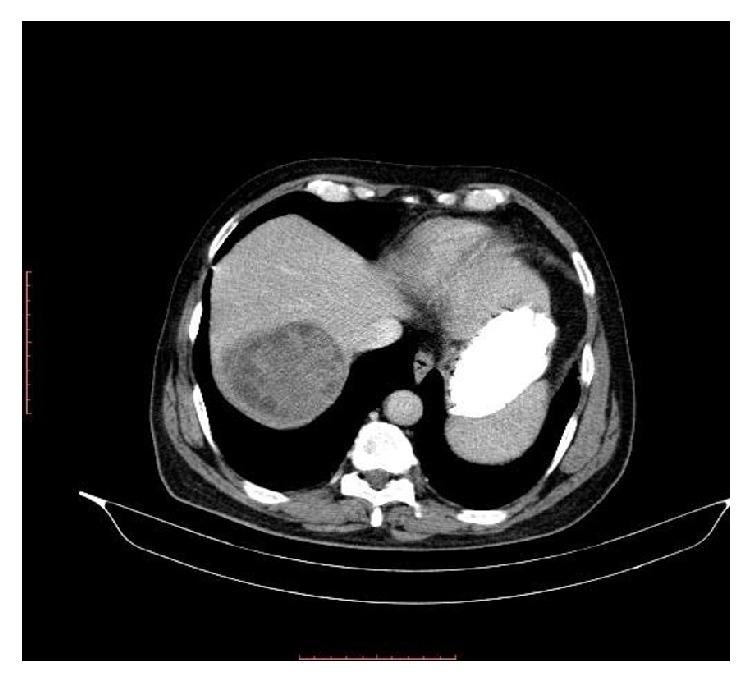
Hydatid cyst in right lobe liver on CT scan.
